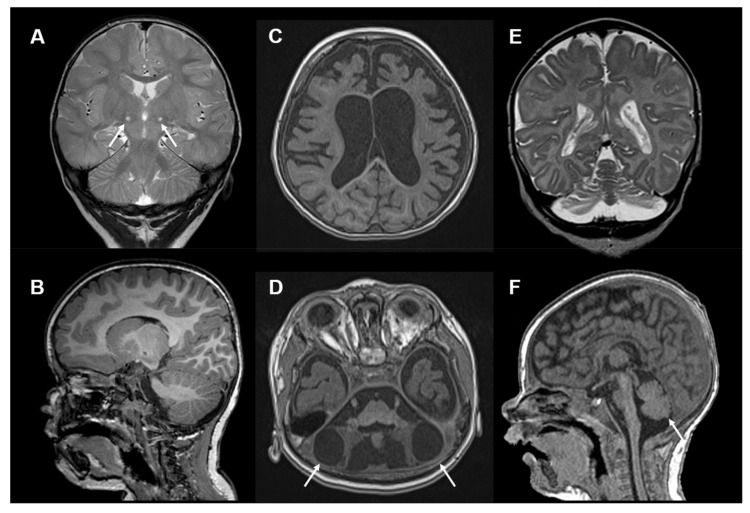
Neuroimaging in COQ4 deficiency: (A) Brain MRI, T2-weighted, coronal images of a 15-month-old girl with COQ4 deficiency. Images show bilateral circumscribed lesions in the subthalamic nuclei (white arrows). (B) Sagittal T1-weithed MRI images show no cerebellar lesions. (C) Brain MRI, T1-weighted, axial images of an 18-month-old girl with COQ4 deficiency showing global brain atrophy. (D) Brain MRI, T1-weighted, axial images of the same child showing cerebellar degeneration with bilateral large cysts within the cerebellar hemispheres (white arrows). (E,F) T2-weighted, coronal and sagittal images and of a 2-month-old girl with COQ4 deficiency showing cerebellar hypoplasia (white arrow). Other MRI images of these individuals were published previously [30].
